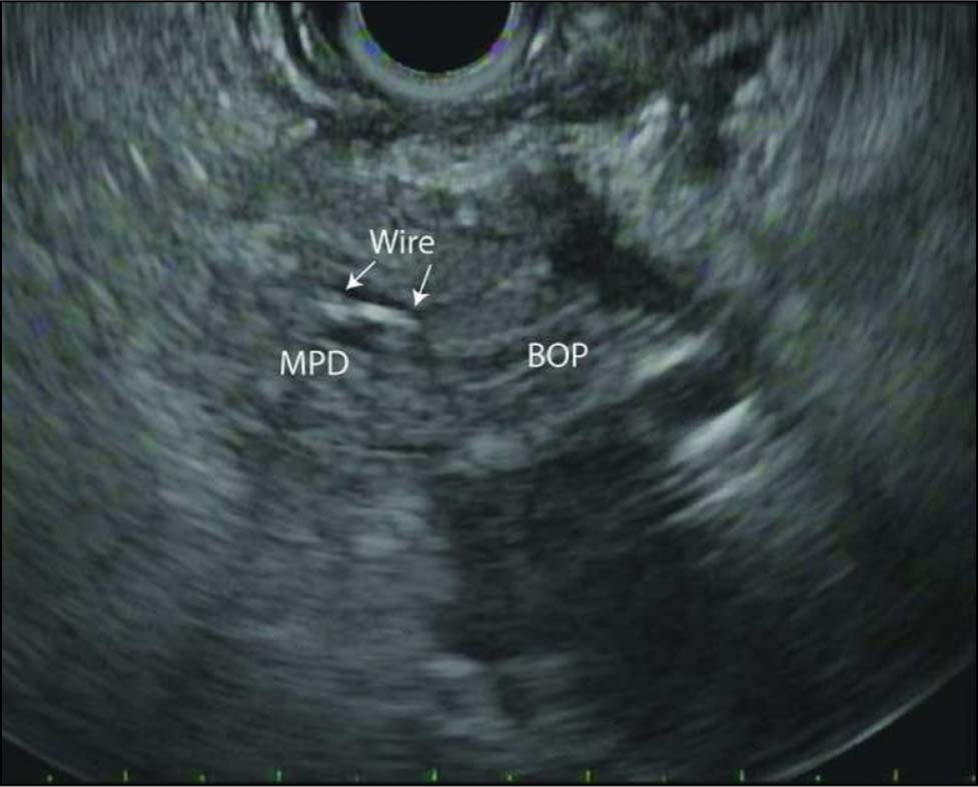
Endoscopic ultrasound showing the retained wire sheath (arrows) within the lumen of the main pancreas duct. MPD, main pancreas duct.
